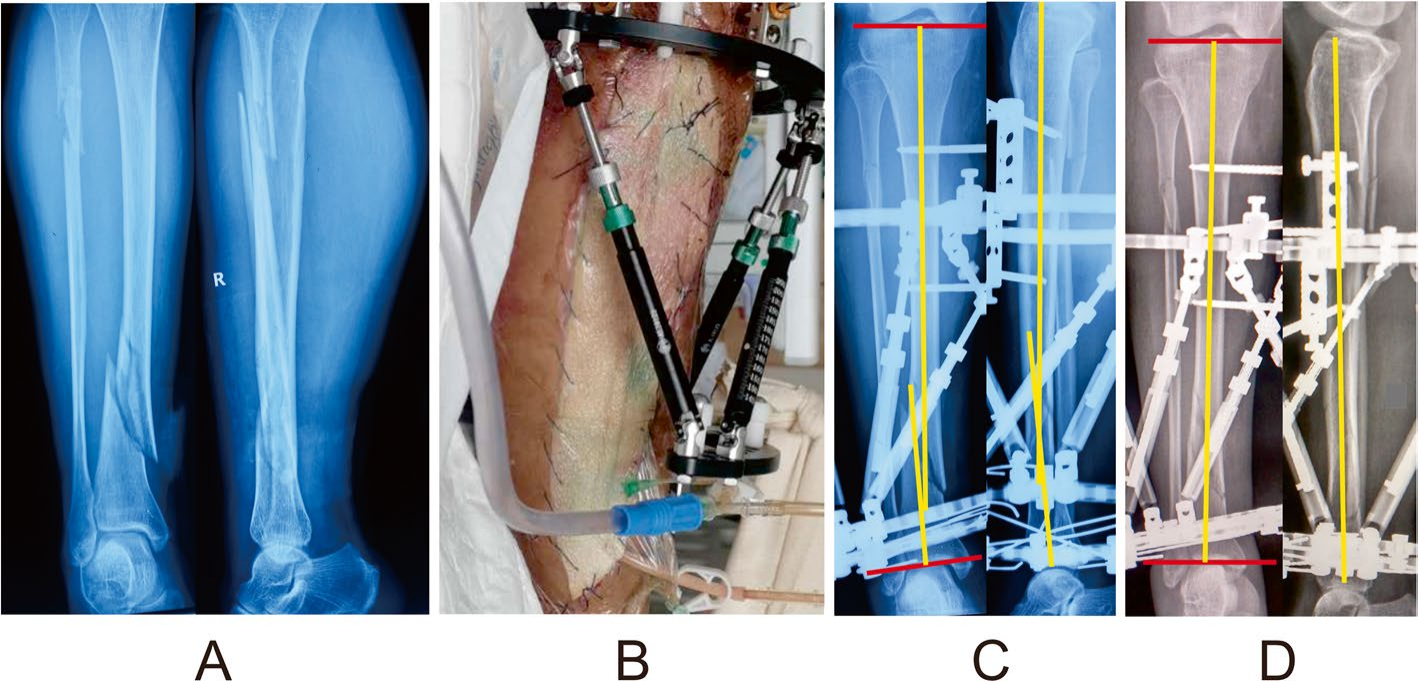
Images of a 39-year-old man with multidimensional deformities in tibia and fibula caused by a road traffic accident and treated by the HEF. a Posttraumatic radiographs. b Patient with compartment syndrome resolved by fasciotomy combing with vacuum sealing drainage technique. c Radiographs immediately after the operation, manifesting varus and flexion residual deformities that needed to be corrected. d Radiographs after final correction, showing satisfactory alignment
